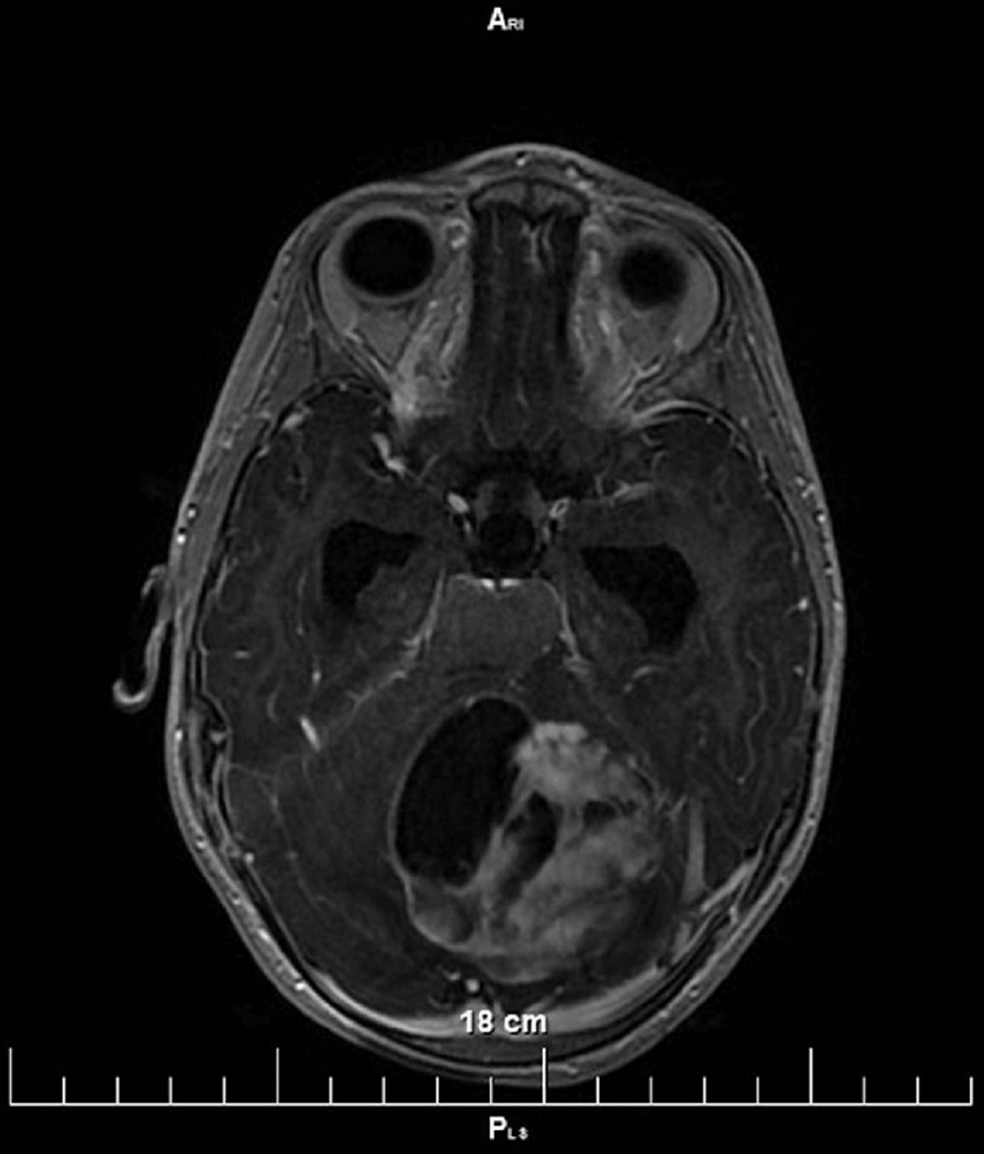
AX 3DT1 native MRI of the brain showing a cystic tumor formation in the left cerebellar hemisphere AX 3DT1: axial three-dimensional longitudinal relaxation time; MRI: magnetic resonance imaging
